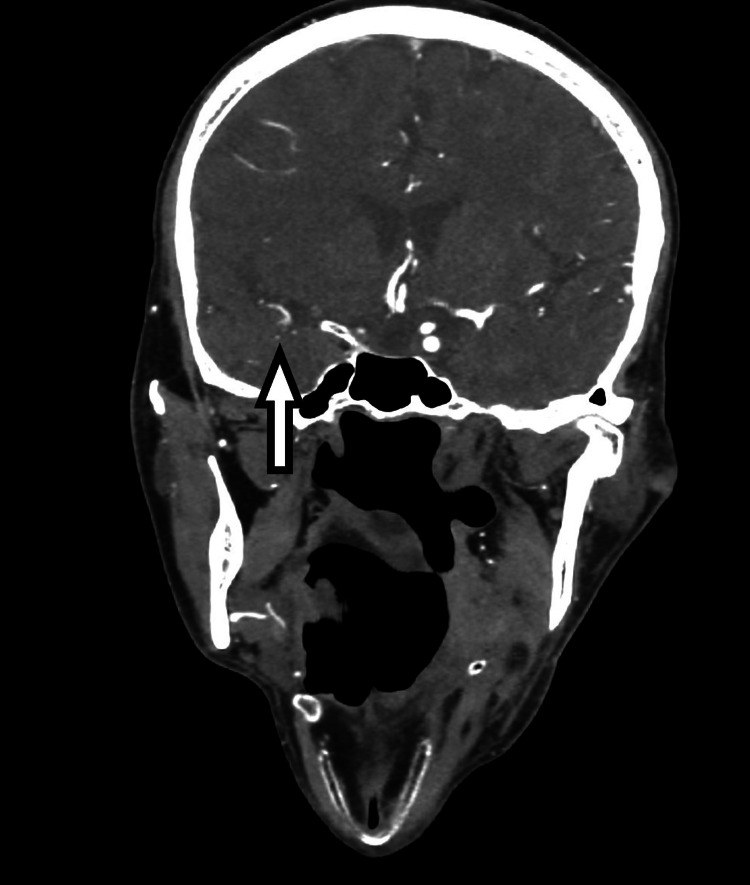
Coronal reconstruction image from computed tomography angiography (CTA) demonstrating tandem occlusion of the right middle cerebral artery M2 branches (white arrow).
